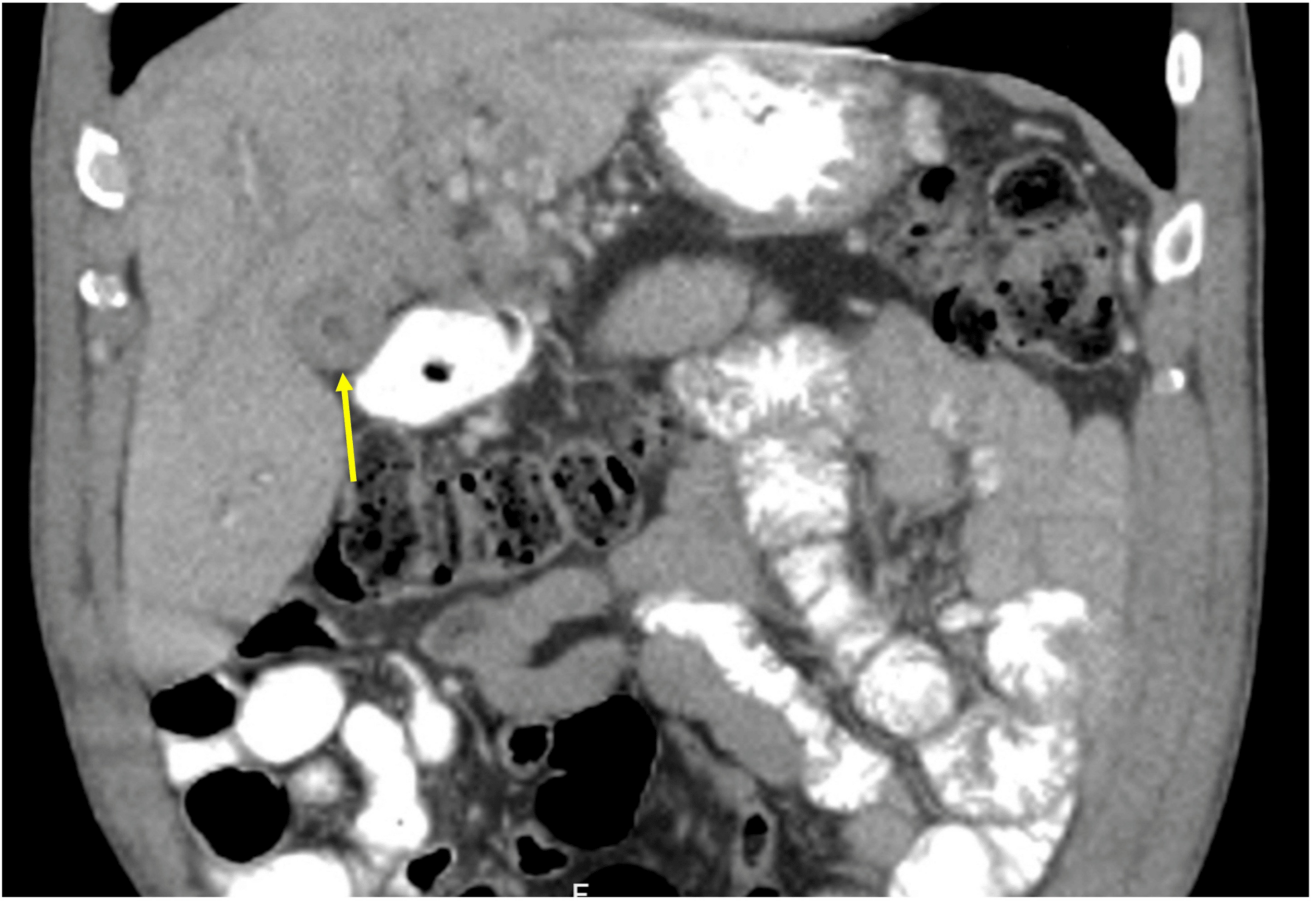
Computed tomography of nonspecific gallbladder wall thickening (yellow arrow).
